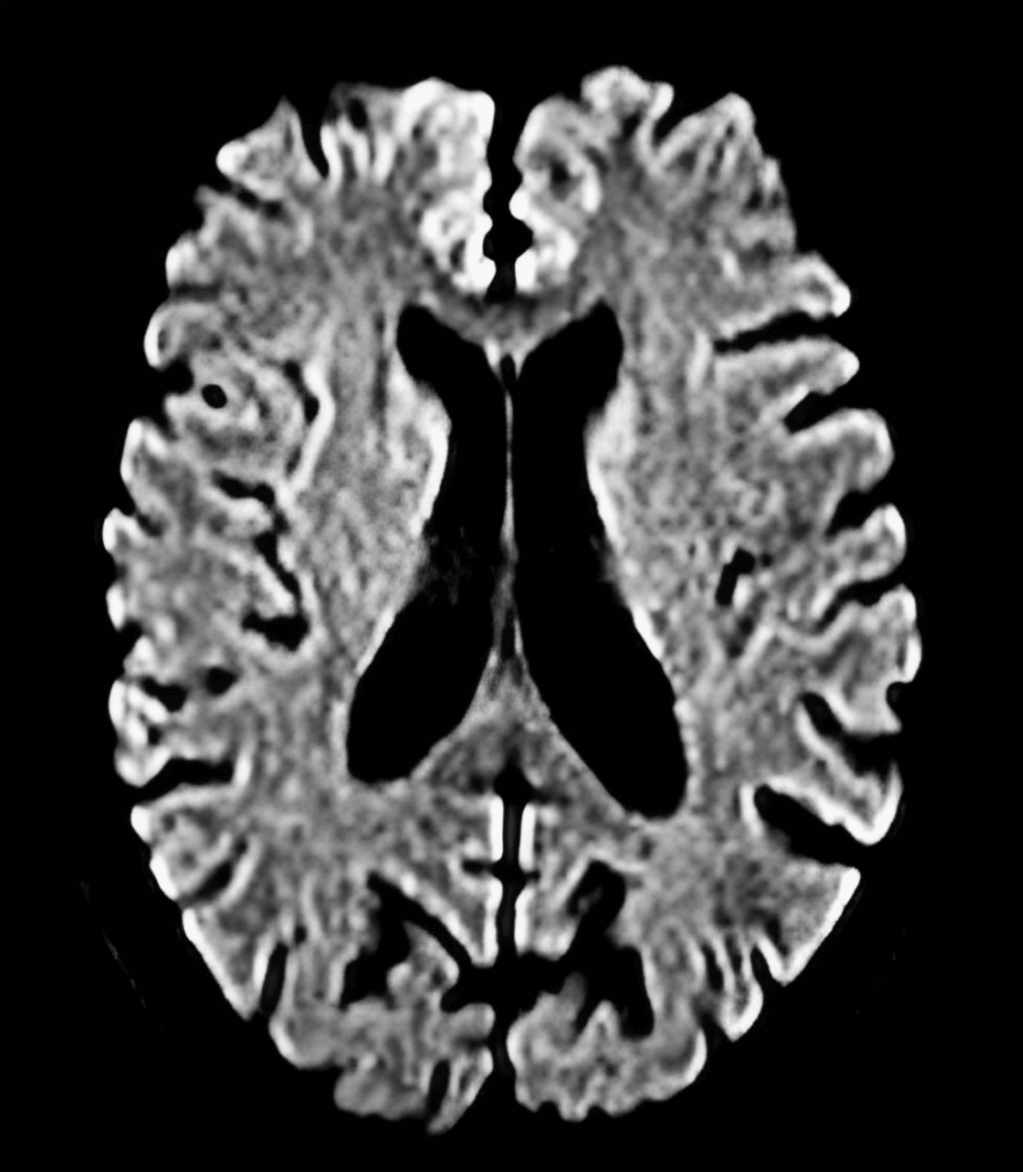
Brain MRI (DWI sequence) showing no evidence of acute ischemia Diffusion-weighted MRI performed four hours after the onset of visual loss demonstrates no restricted diffusion in the occipital lobes or elsewhere, excluding acute ischemic stroke. The normal appearance supports the diagnosis of transient contrast-induced cortical dysfunction rather than infarction.
